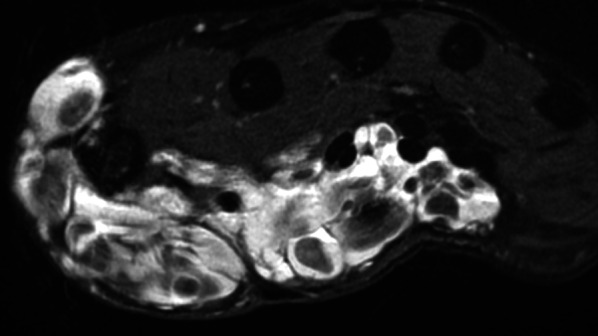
Secondary intravascular papillary endothelial hyperplasia in a 45-year-old woman taking the aspect of a low-flow vascular malformation affecting the thenar eminence. Axial T2-weighted MR image showing a polylobular mass with multiple cavernous lakes containing phleboliths
